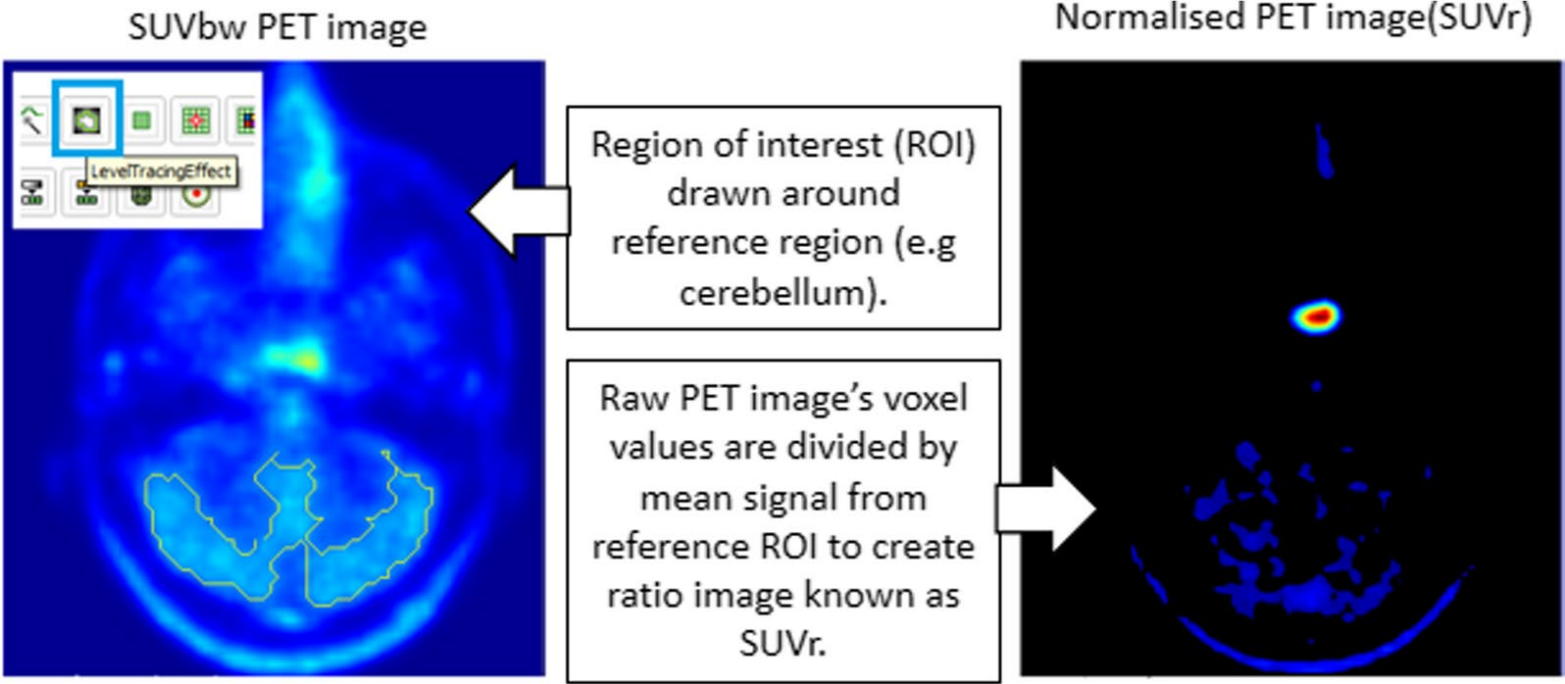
Normalization of PET images. ROI drawn around the reference region (cerebellum shown here) on one slice of the PET image. The PET images are divided by the mean signal from the reference ROI to create a ratio image known as standardized uptake value ratios (SUVrs)
